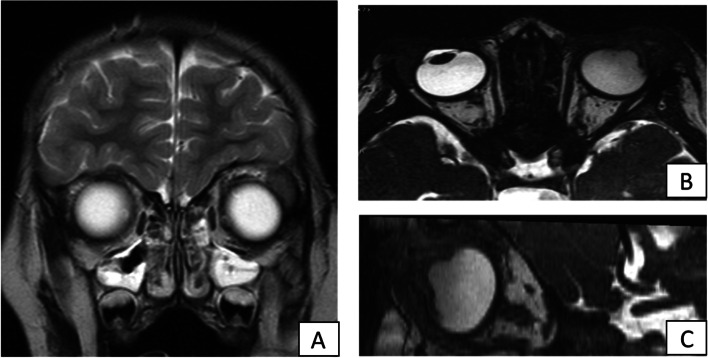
A, B and C: Brain MRI with Optic Nerves study, showing severe involvement of the left eye
